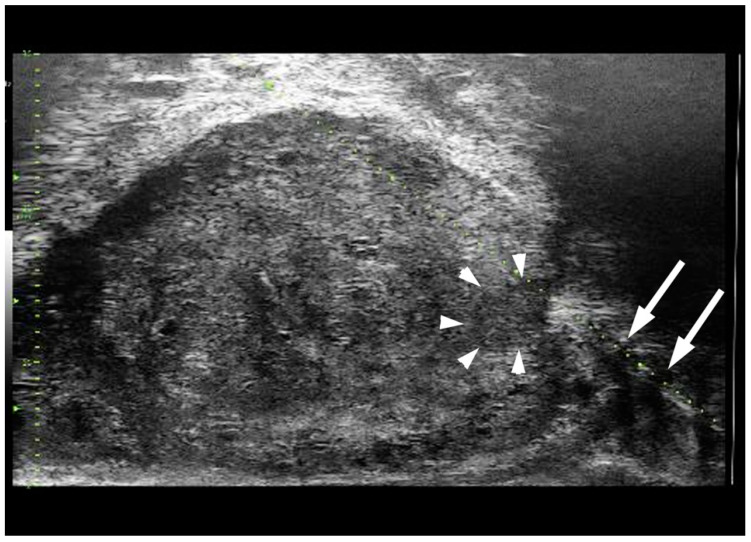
PRIMUS 4 nodule (arrowheads) identified in the apical peripheral zone on microUS with the biopsy needle (arrows). Real time visualization of the nodule allows accurate targeting without MRI/microsUS fusion.
